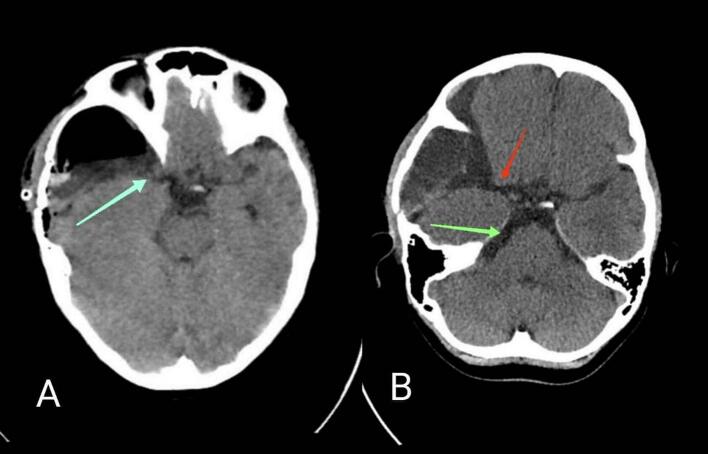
Postoperative and follow-up CT images of a third ventricular arachnoid cyst treated with endoscopic cystocisternostomy. A) Immediate postoperative CT scan showing the site of fenestration (blue arrow) between the arachnoid cyst and the basal cisterns. B) Follow-up CT obtained several months later demonstrates a patent communication (red arrow) between the cyst cavity and the basal cisterns, with clear visualization of cerebrospinal fluid flow in the basal cisterns (green arrow).
